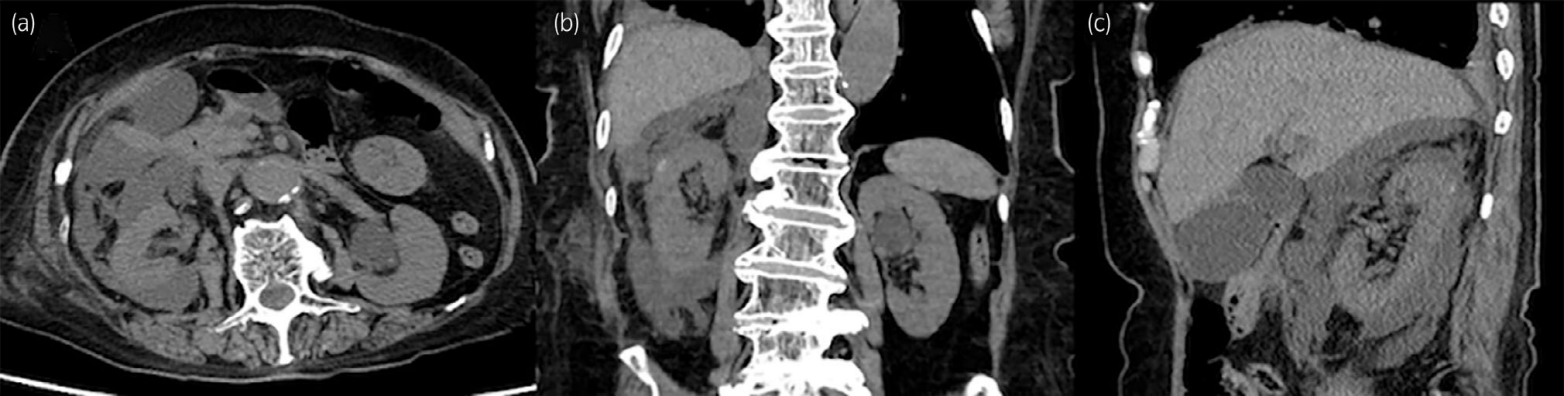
CT revealed fluid accumulation in the retroperitoneum and an increased concentration of right perirenal fatty tissue. (a) Axial section, (b) coronal section, (c) sagittal section.
